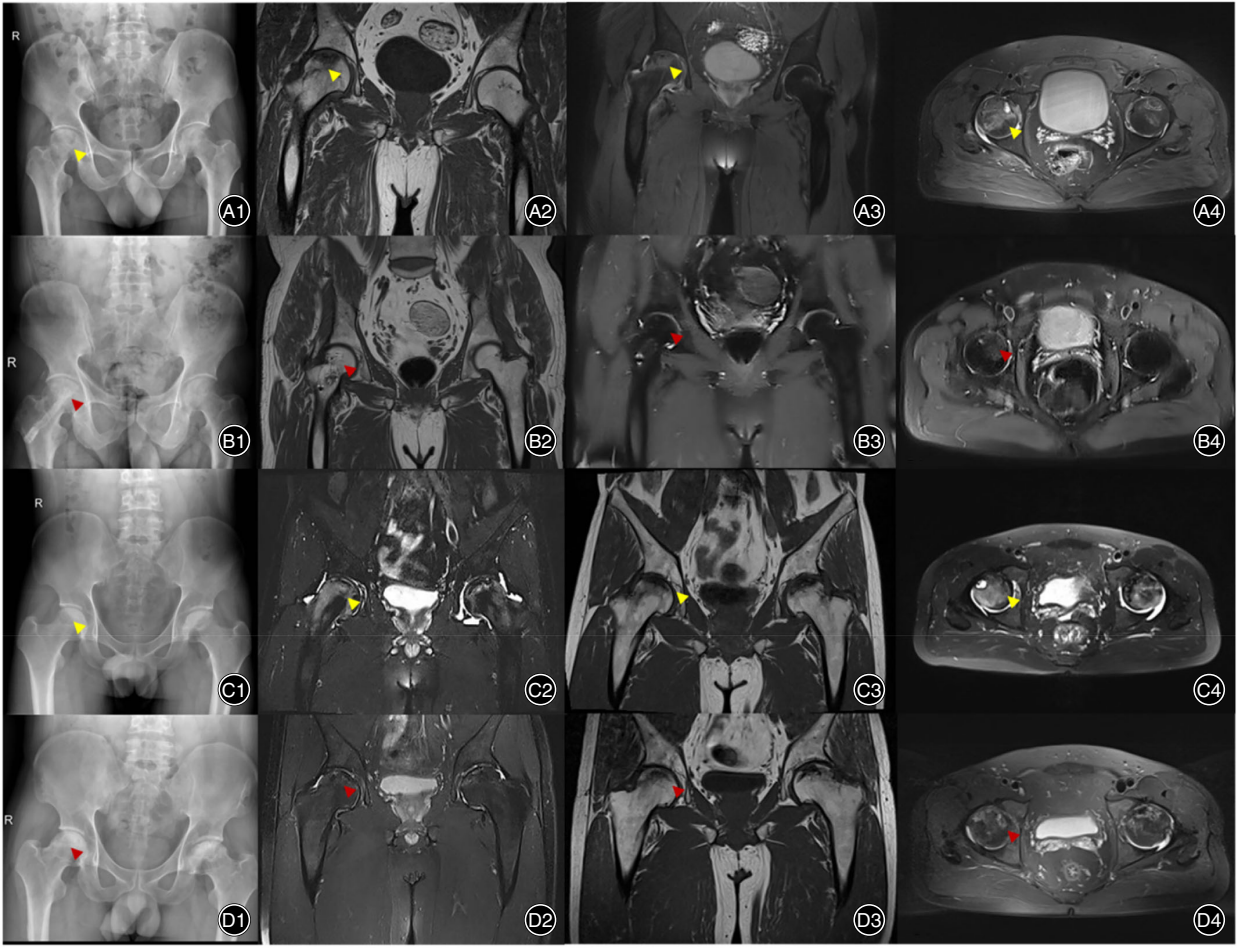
Preoperative and last follow‐up magnetic resonance imaging (MRI) images for patients who received arthroscopically assisted “light bulb” core decompression (CD) and bone graft implantation.
